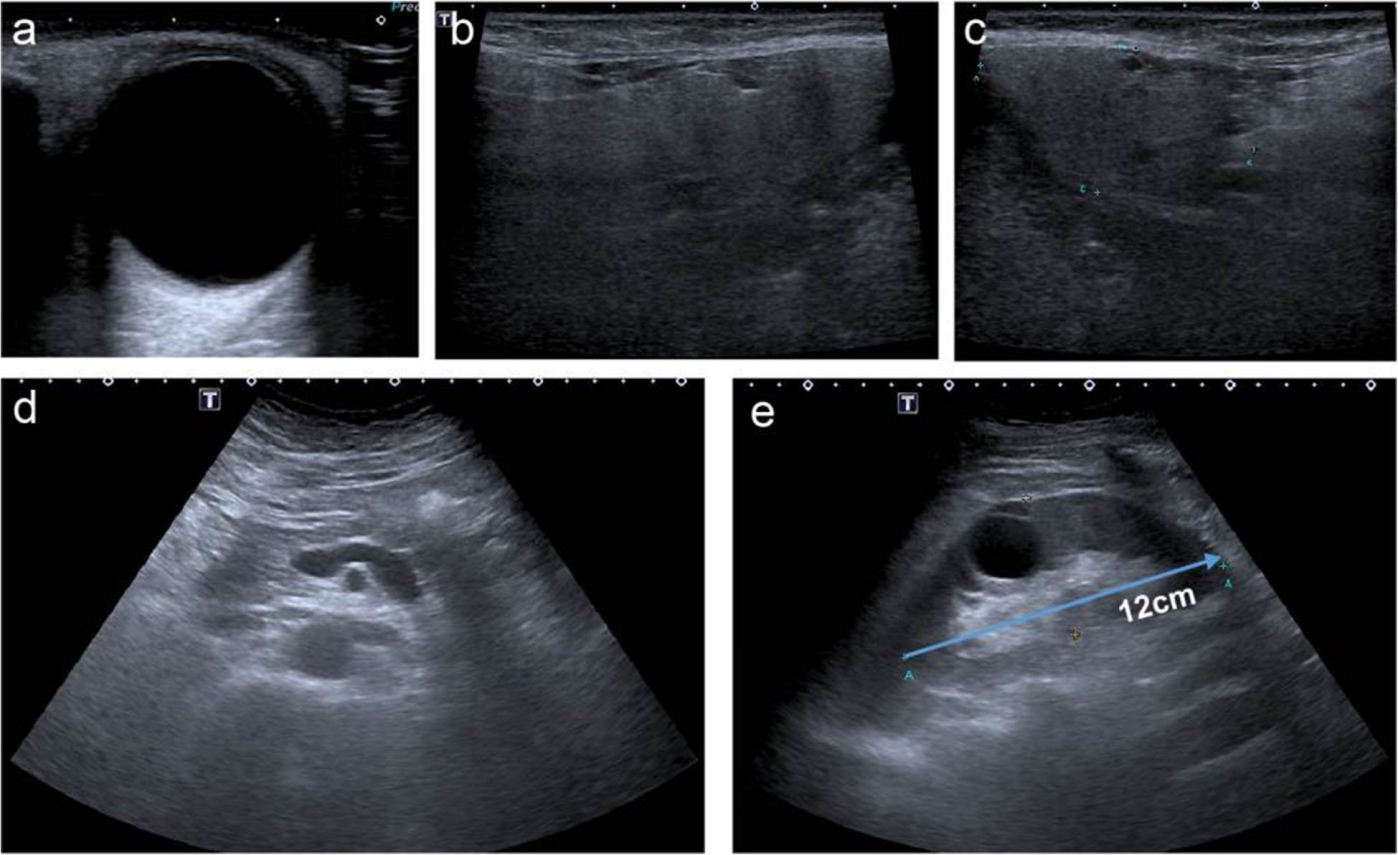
Ultrasound images of extrarenal and renal manifestations. No enlargement of lacrimal gland (a), parotid gland (b), submandibular glands (c), pancreas and retroperitoneal fibrosis (d). Normal size kidney and renal cortex thickness and ureteral inflammatory pseudotumor (e) at initial admission
